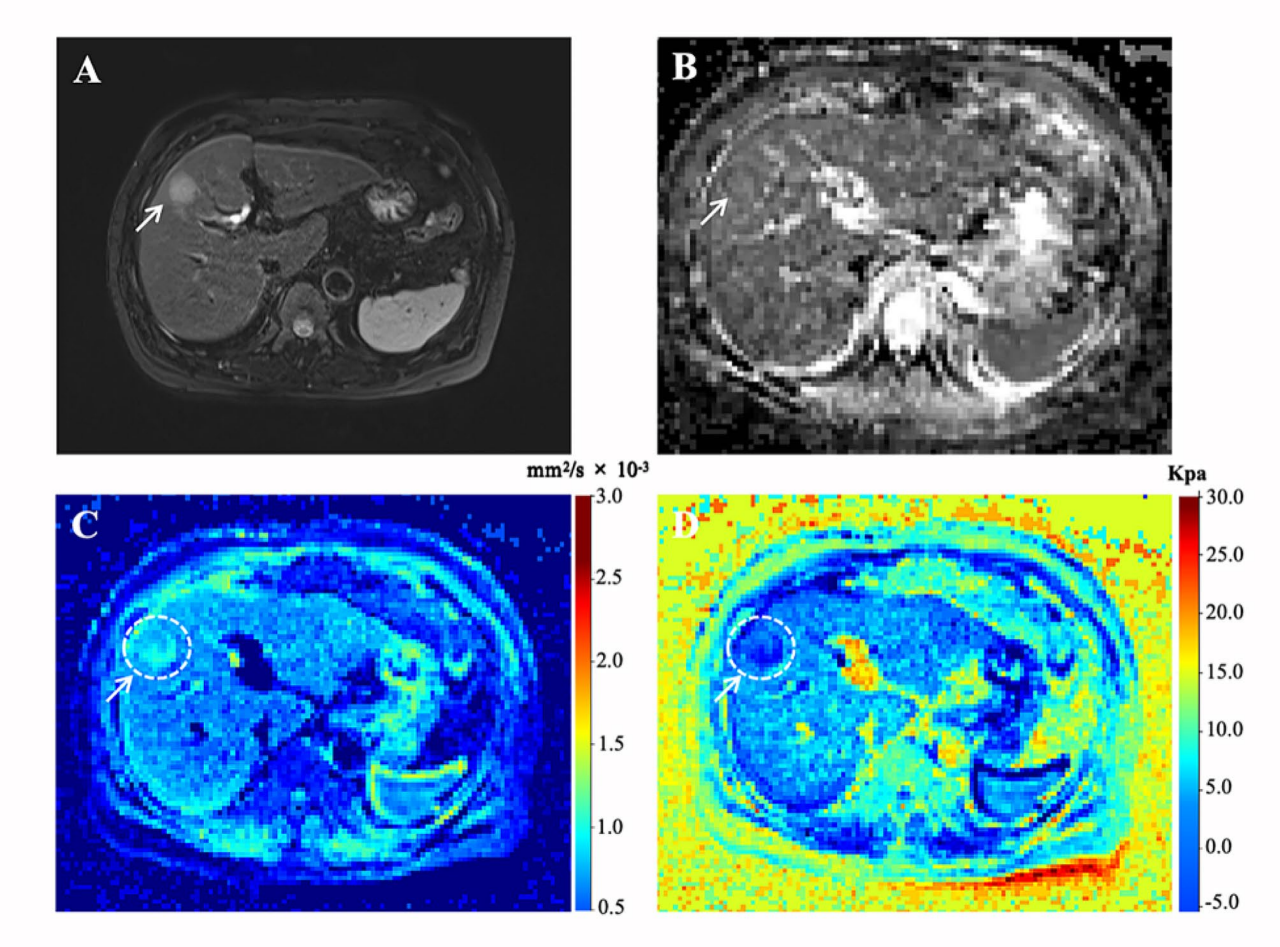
Representative MR images of a 60-year-old woman with pathologically verified HCC without recurrence during a 5-year follow-up. (A) Axial T2-weighted image shows a hyperintensity mass (arrow) on segment VIII of liver. (B-D) ADC, sADC, and μ_diff_ maps showing that the mean ADC, sADC, and μ_diff_ values of the tumor (arrow) were 1.35 × 10^− 3^ mm^2^/s, 0.94 × 10^− 3^ mm^2^/s, and 1.99 kPa, respectively. ADC, apparent diffusion coefficient; sADC, shifted apparent diffusion coefficient; *μ*_*diff*_, DWI-based virtual shear modulus
